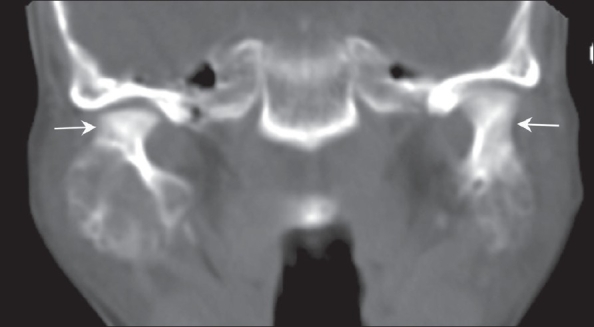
Coronal CT scan reveals sparing of both mandibular condyles (arrows)
